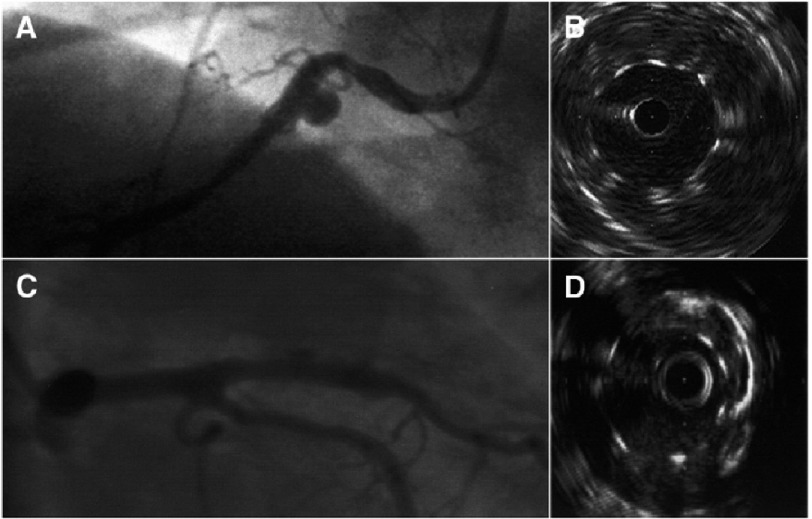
Angiographic and IVUS images of coronary artery aneurysm. (A and B) Coronary artery aneurysm detected by coronary angiography and intravascular ultrasound (IVUS) 8 months after bare-metal stent implantation. (C and D) Coronary artery aneurysm detected with coronary angiography and IVUS 3 months after drug-eluting stent implantation. *From Aoki et al.*^16^
